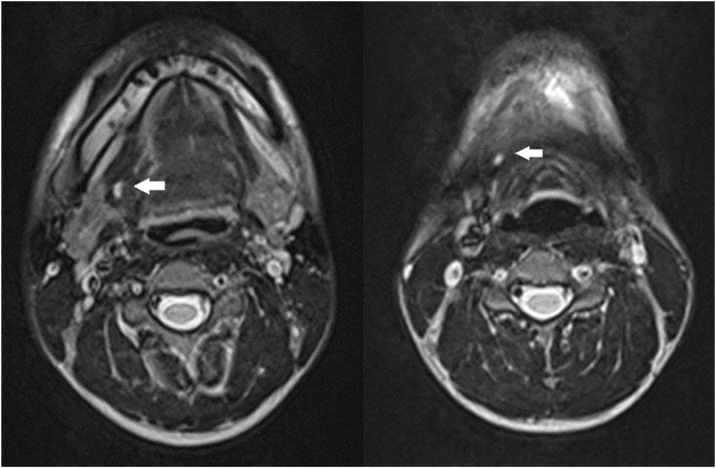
T2-weighted magnetic resonance imaging of the neck. The luminal structure extends from the surface of the right lateral anterior neck to the right oral floor.
